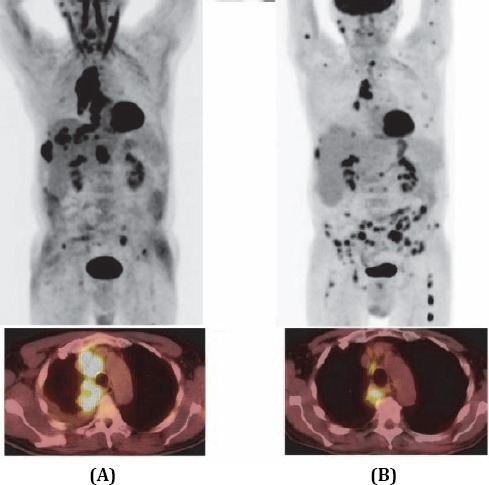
^18^F-FDG PET/CT scan before and after therapy. A. At the initial staging, B. Response to the therapy of primary tumor and locoregional disease
